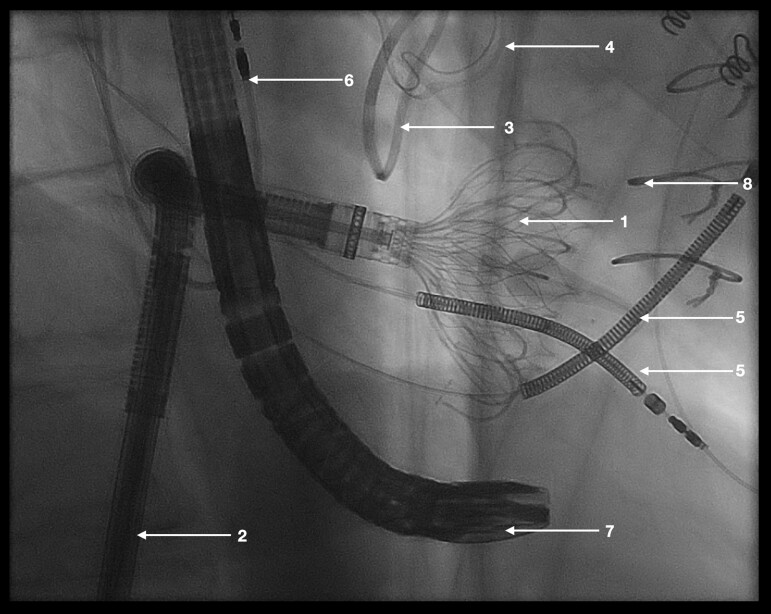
Fluoroscopy during implantation of the EVOQUE prosthesis shows a fluoroscopic image of the implantation process. 1—partially deployed EVOQUE prosthesis; 2—EVOQUE delivery system; 3—Carpentier–Edwards Physio II annuloplasty ring; 4–23 mm Carpentier–Edwards Magna Ease-Bioprosthesis; 5—right ventricular implantable cardioverter-defibrillator leads; 6—right atrial pacemaker lead; 7—transoesophageal echocardiography probe; 8—sternal cerclage.
